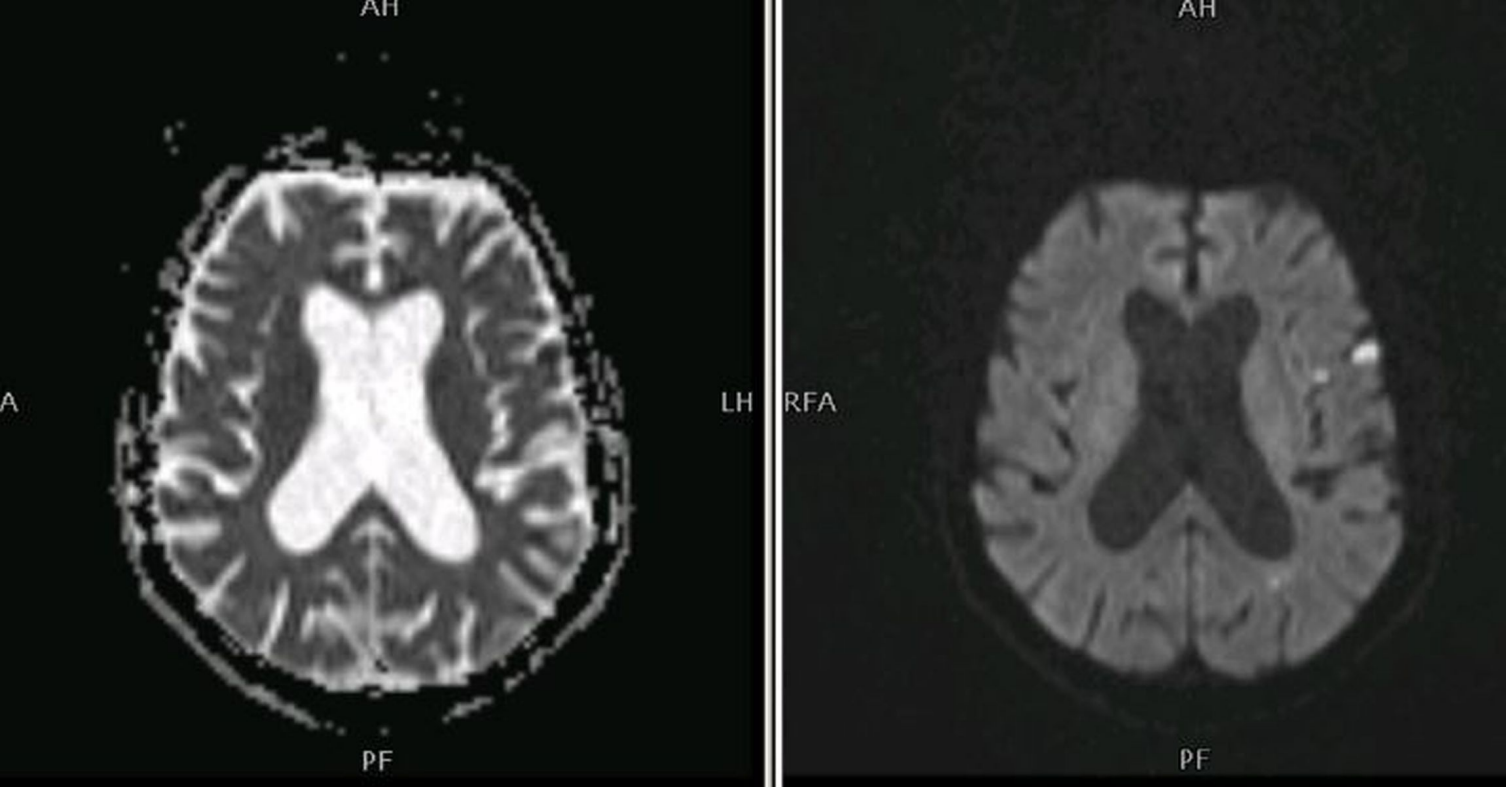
Cortical infarct pattern Bright diffusion-weighted imaging (DWI) and low apparent diffusion coefficient (ADC) images showing a small cortical stroke. The patient was hypertensive and had a score of more than 60 on the Barthel index.
